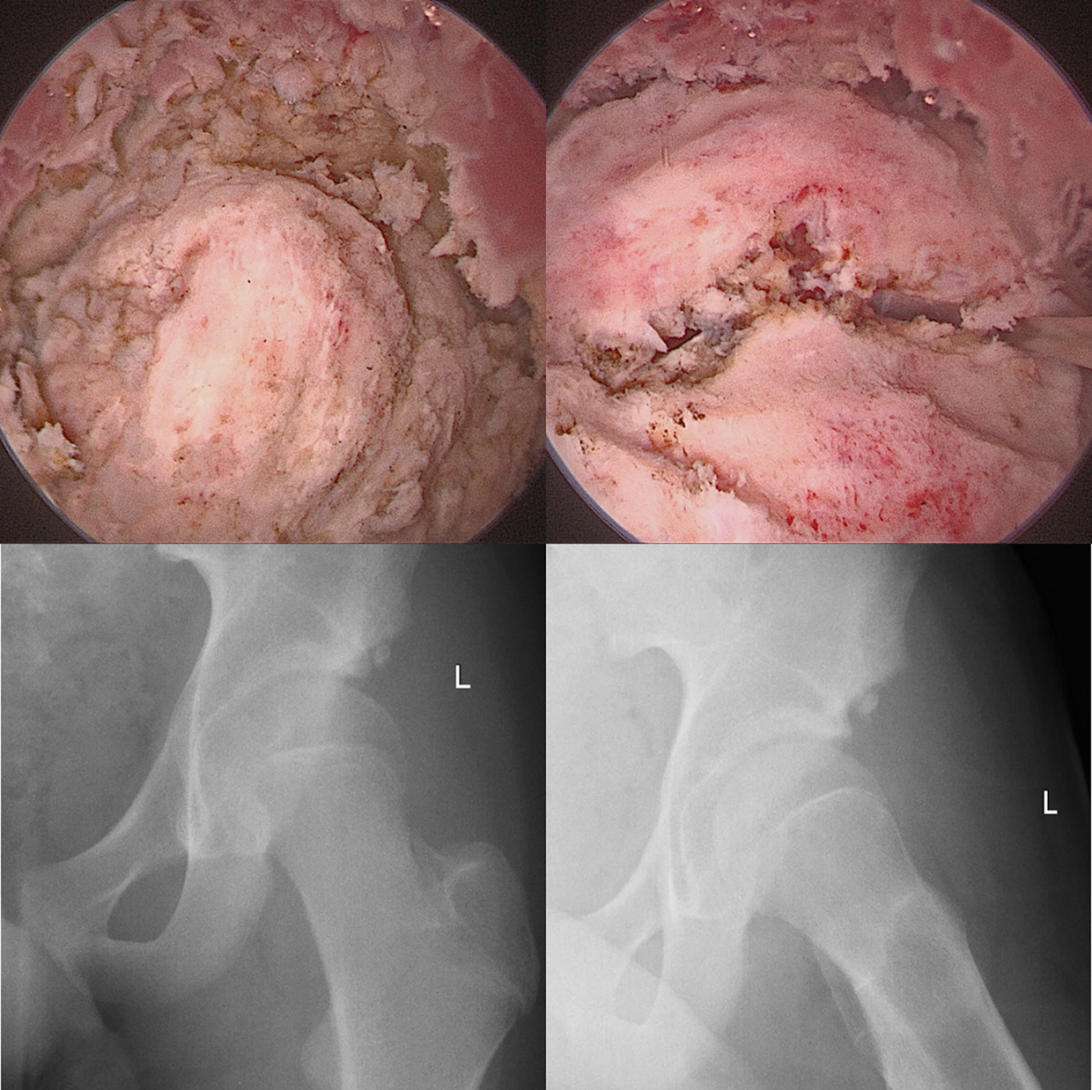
Arthroscopic images of the heterotopic ossification that occurred in the rectus femoris muscle which was excised and extracted via an incision made on the anterolateral aspect of the thigh, and post-operative radiograph which shows that the heterotopic ossification lesion was cleared completely.
